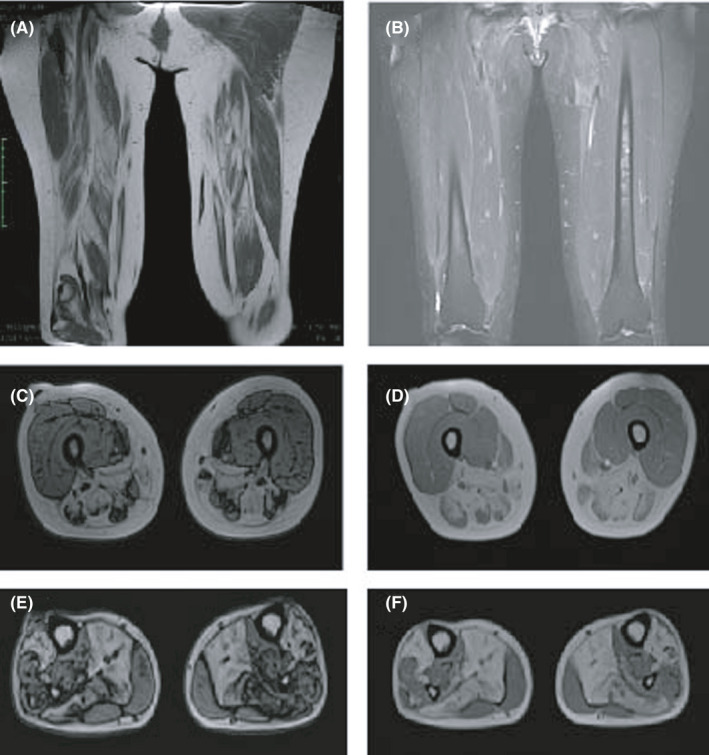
MRI in patient No.5 revealed increased signal intensity of the femur shaft and hamstrings; MRI in patient No.6 revealed pronounced fatty infiltration along with muscle atrophy in the posterior and internal compartments of the thigh muscles and lower leg muscles. (A and B), coronal axial MRI image of thigh muscles in patient No.5. (C and D), transverse axial image of thigh muscles in patient No.6. (E and F), transverse axial image of lower leg muscles in patient No.6
